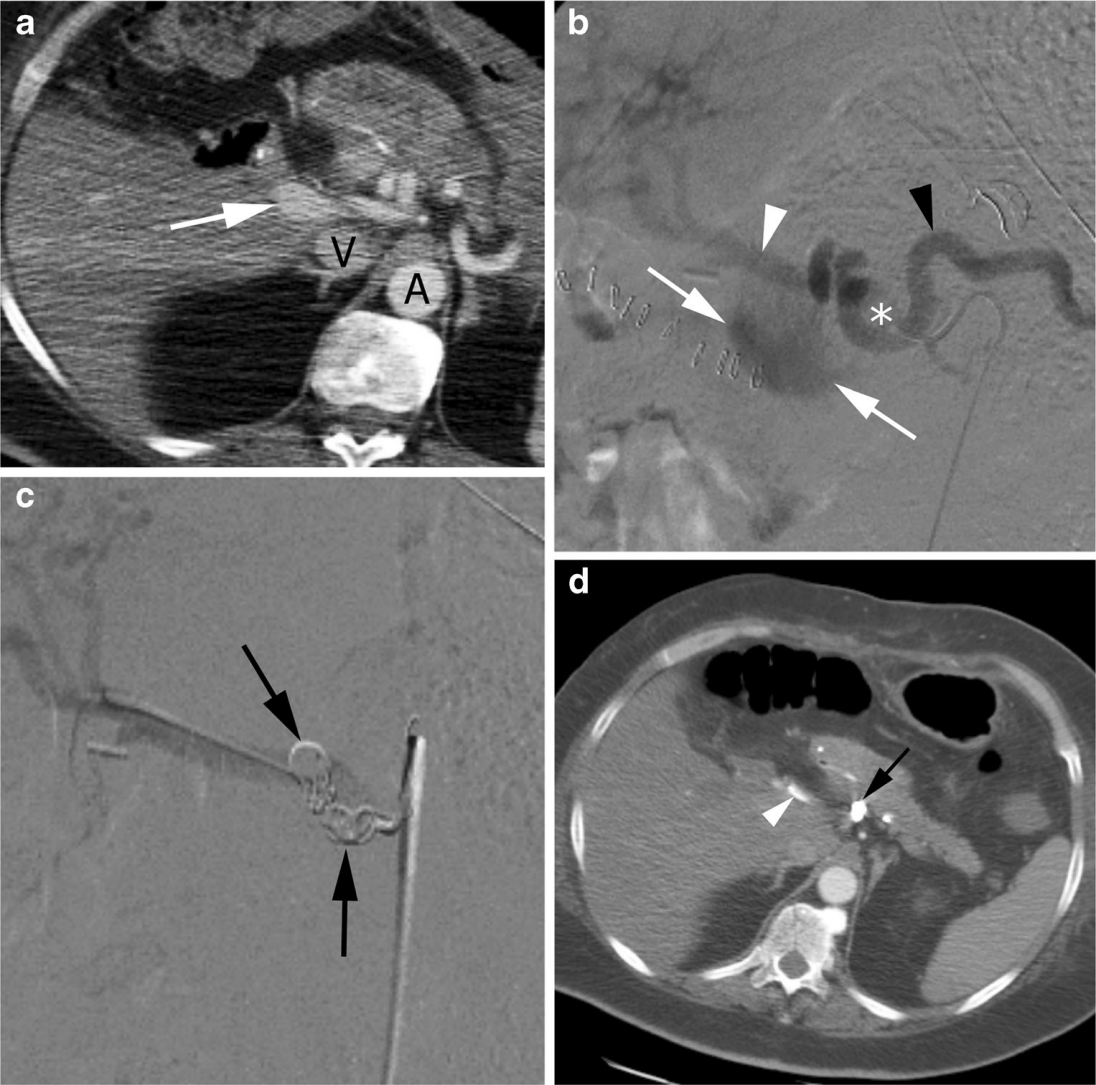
Arterial embolisation of a post-surgical haemorrhage. a CT scan showing contrast extravasation (*arrow*) from the celiac trunk at the level of the bifurcation between hepatic and splenic artery; *V* inferior vena cava, *A* abdominal aorta. b Angiographic appearance of the active bleeding with contrast extravasation (*arrow*s); *asterisk* celiac trunk, *black arrowhead* splenic artery, *white arrowhead* hepatic artery. c Angiography after embolisation with coils (*arrows*) demonstrating absence of contrast extravasation at the level of previous bleeding. d CT scan at 2 years, demonstrating absence of contrast extravasation at the level of previous bleeding and preserved patency of proper hepatic artery (*white arrow*), even in the presence of part of a coil in its lumen (*arrowhead*); *black arrow* clustered metallic coils in the focus of arterial lesion properly embolised
